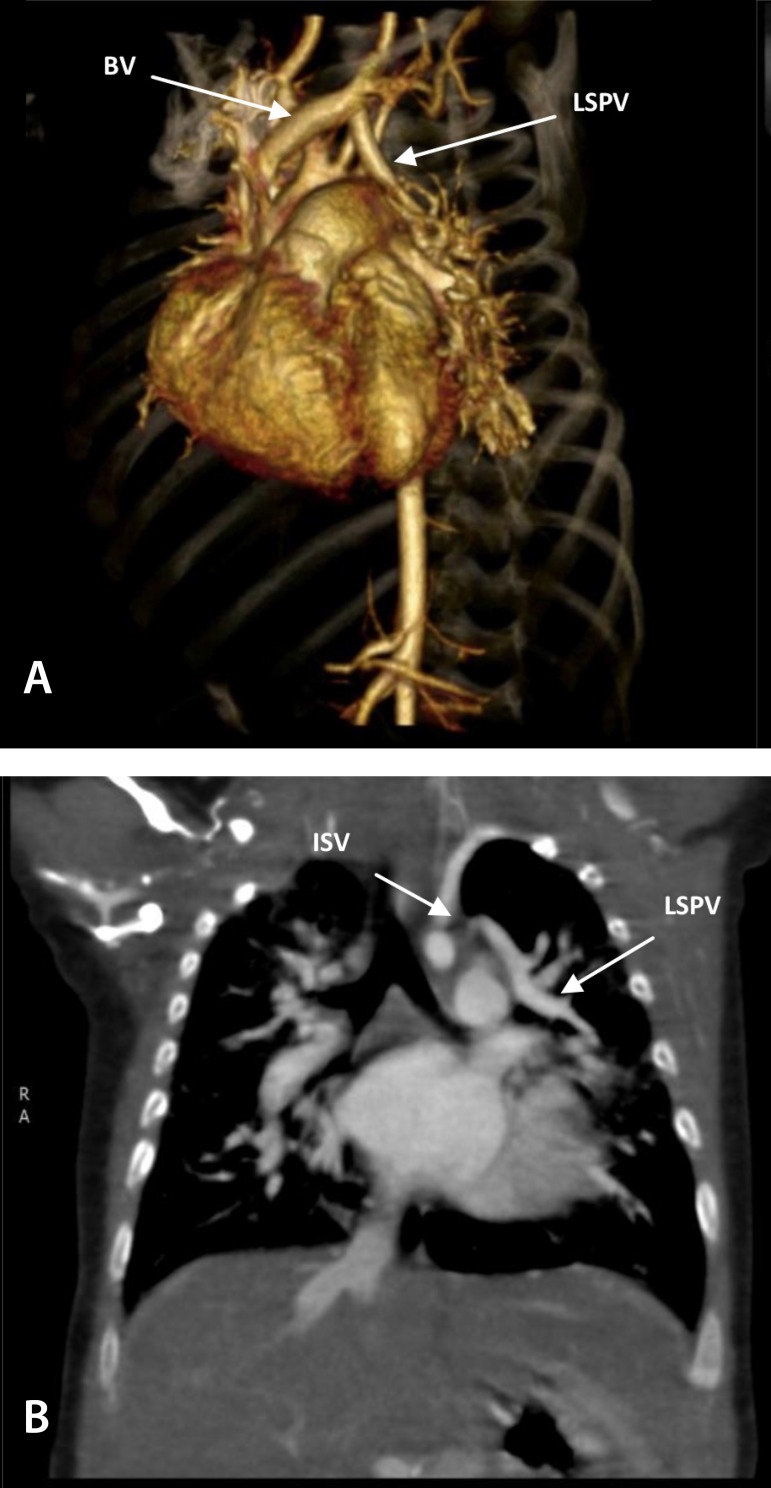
A) 3D volume-rendering computed tomographic angiography showing partial anomalous pulmonary venous connection characterized by LSPV draining directly into BV. B) Small ISV draining in the LSPV before LSPV enters the BV. BV=brachiocephalic vein; ISV=intercostal systemic vein; LSPV=left superior pulmonary vein
